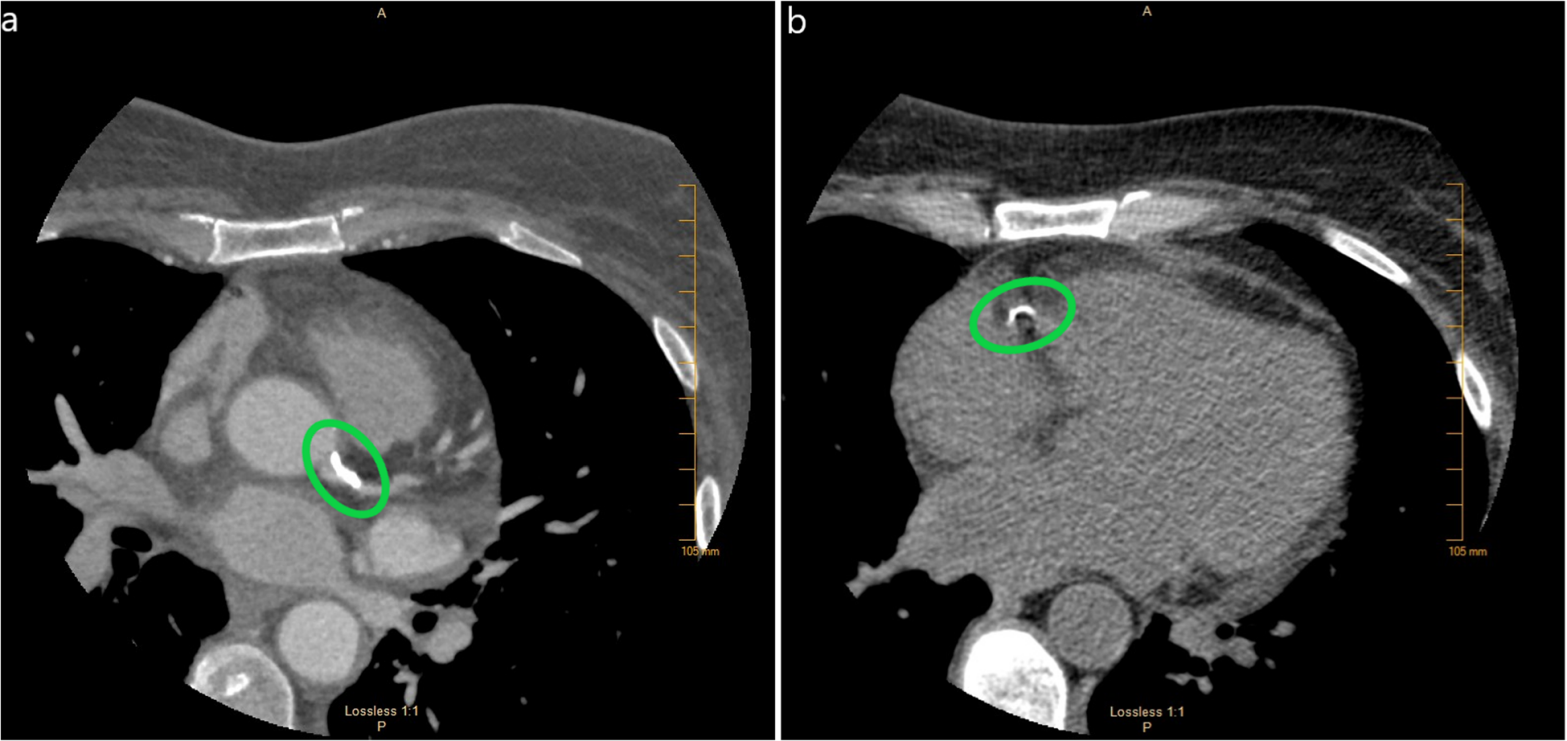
Coronary CT angiogram images showing areas of calcification (green circles). a Left circumflex artery b Right coronary artery
